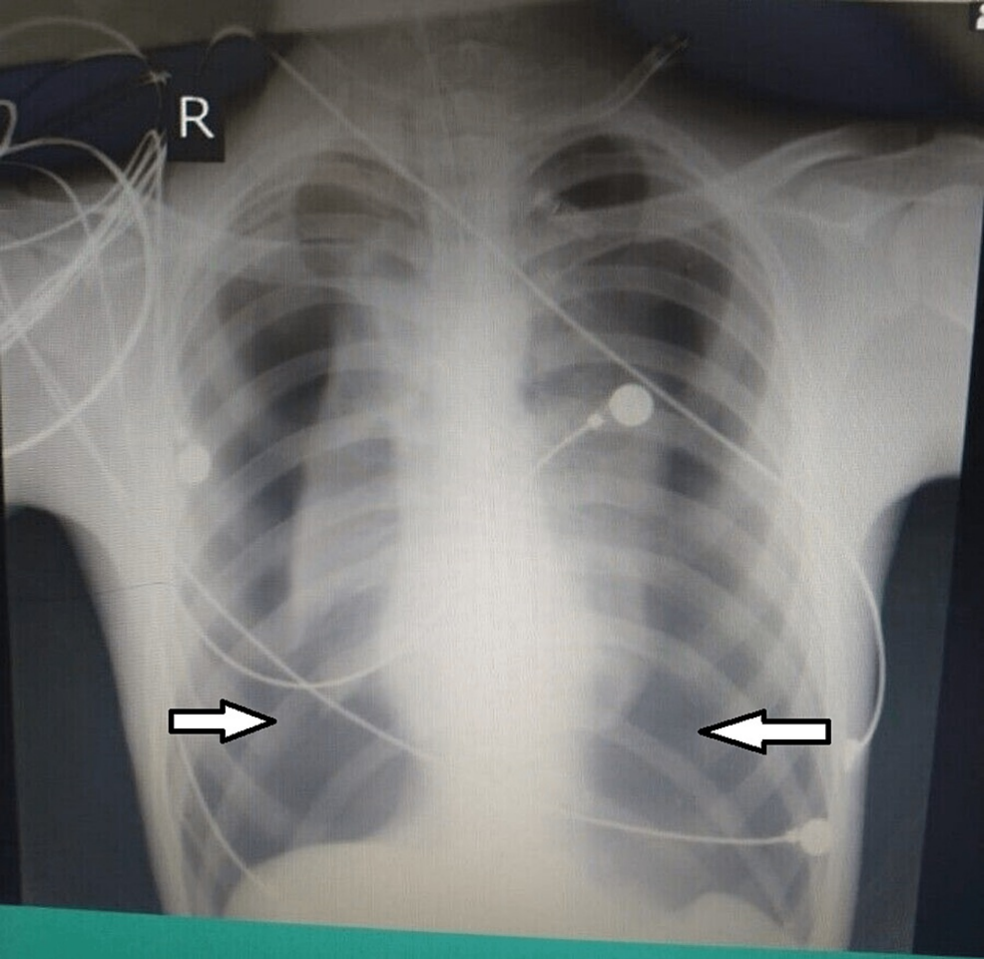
Chest X-Ray showing bilateral pneumothorax
